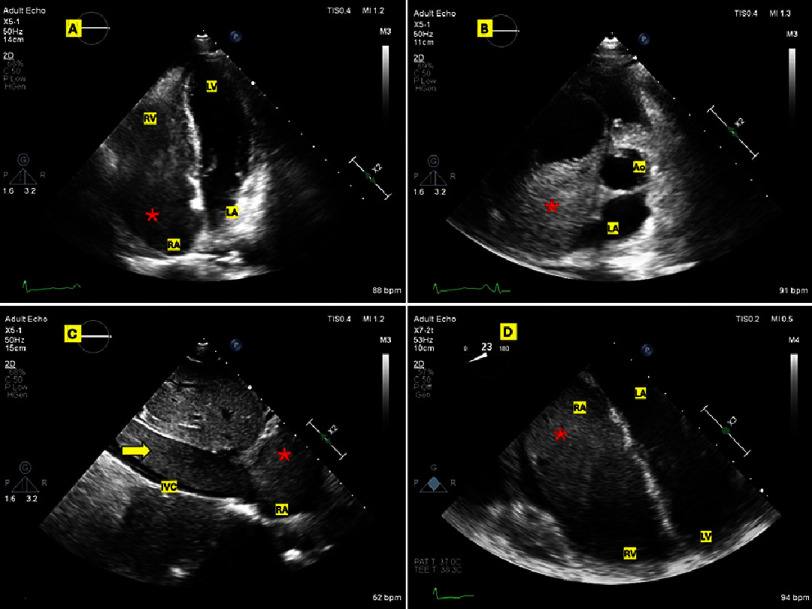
Pre-operative TTE and TEE. (A): TTE A4C view showing the large mass (red asterisk) occupying RA and RV. (B) SAX view with the mass prolapsing from RA to RV. (C) The elongated mass in IVC (red arrow) entering RA. (D) TEE 4-chamber view with the mass nearly filling RA and RV. A4C, Apical 4-chamber; IVC, Inferior vena cava; RA, Right atrium; RV, Right ventricle; SAX, Short axis; TEE, Transesophageal echocardiography; TTE, Transthoracic echocardiography.
